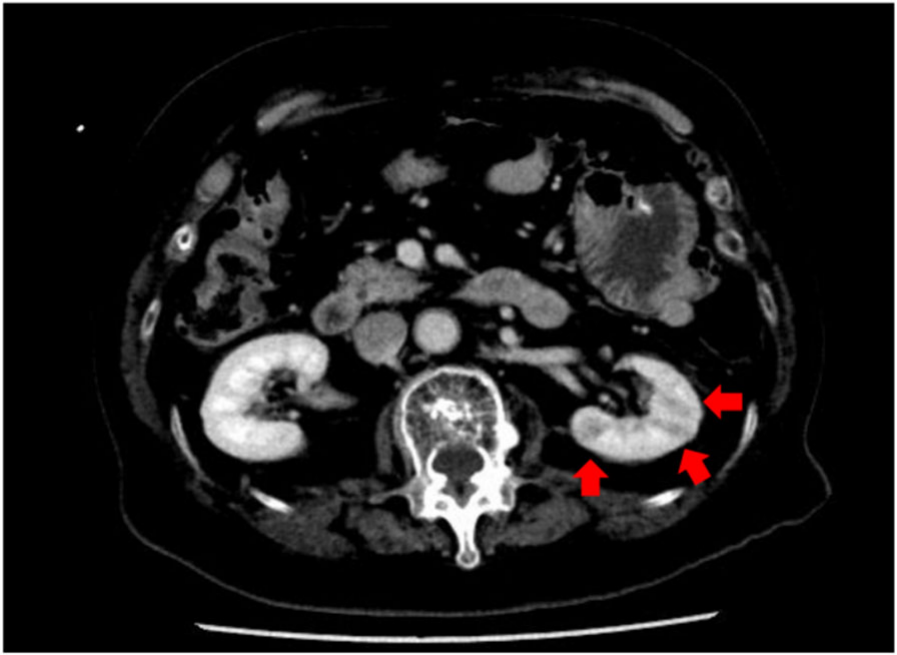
Abdominal computed tomography (CT) on admission. Poor contrast of the left kidney on enhanced CT demonstrating pyelonephritis. red arrows: poor contrast area
